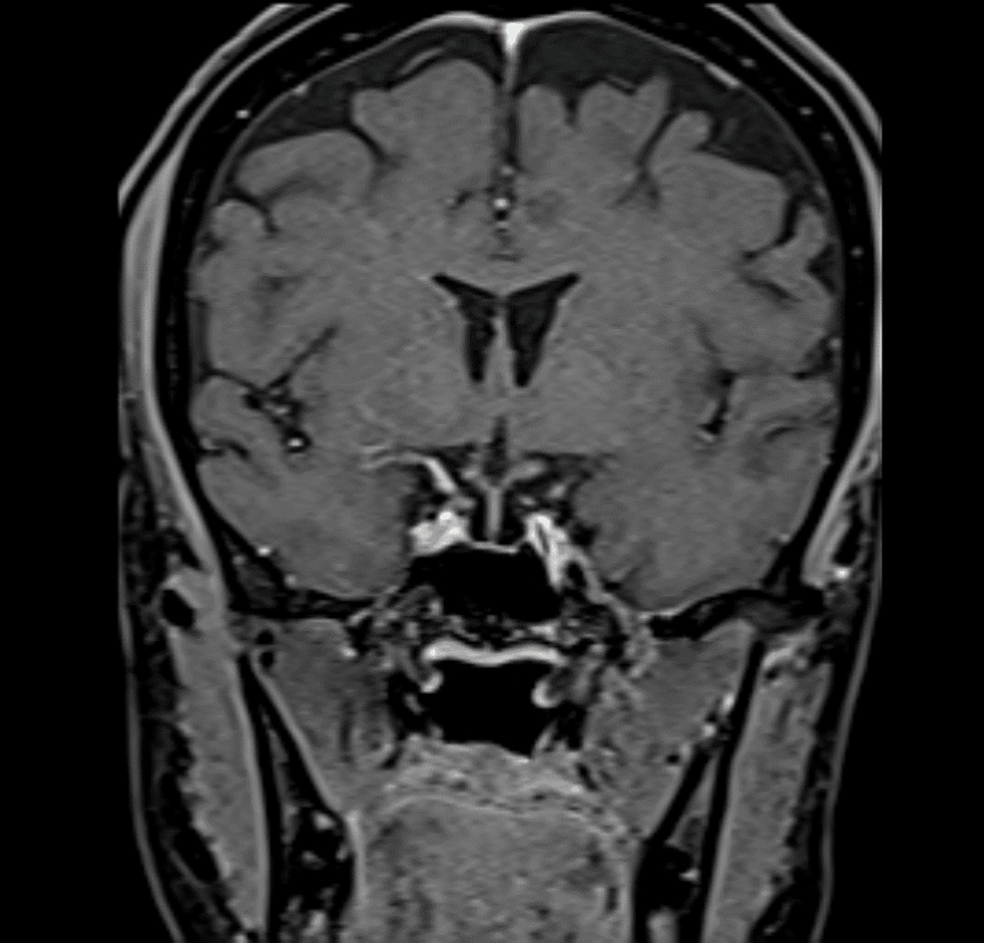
Patient's MRI of the sella turcica T1 coronal MRI of the patient with the finding of an empty sella turcica.
